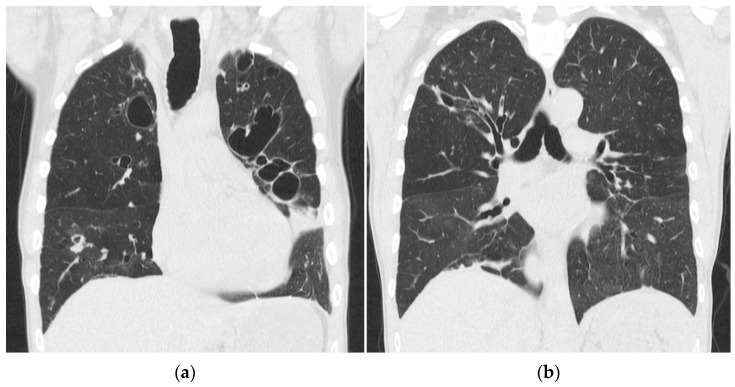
(a,b) CT scan of the chest with coronal reconstruction, showing bilateral pulmonal cysts, bronchiectasis, consolidation and dilation of the trachea, 5 months after admission.
